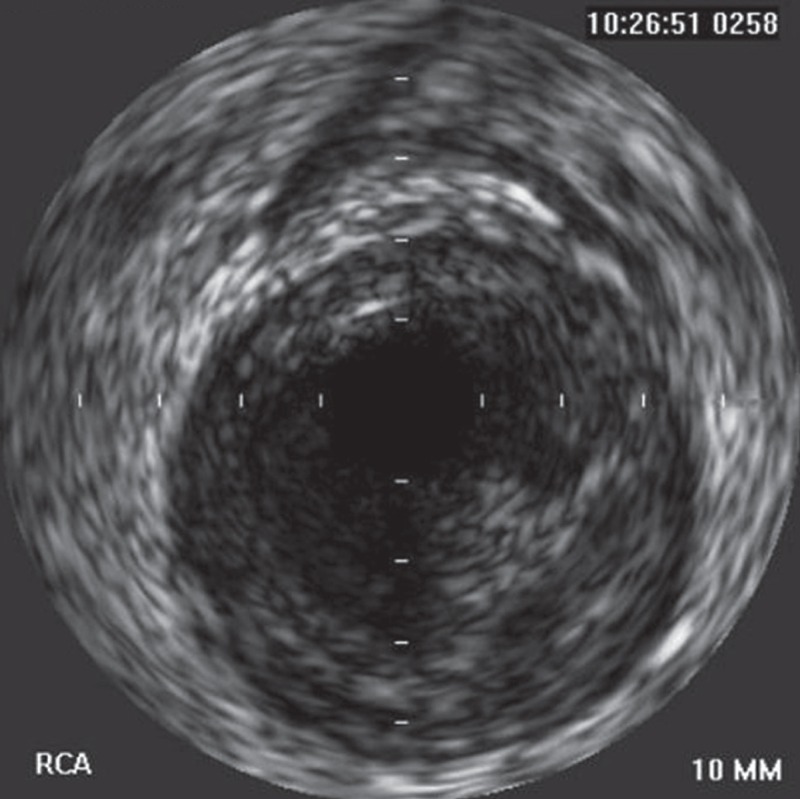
Intravascular ultrasound (IVUS) revealed thrombi still present in the large RCA. The reference diameter of the RCA was 6.2 mm.
